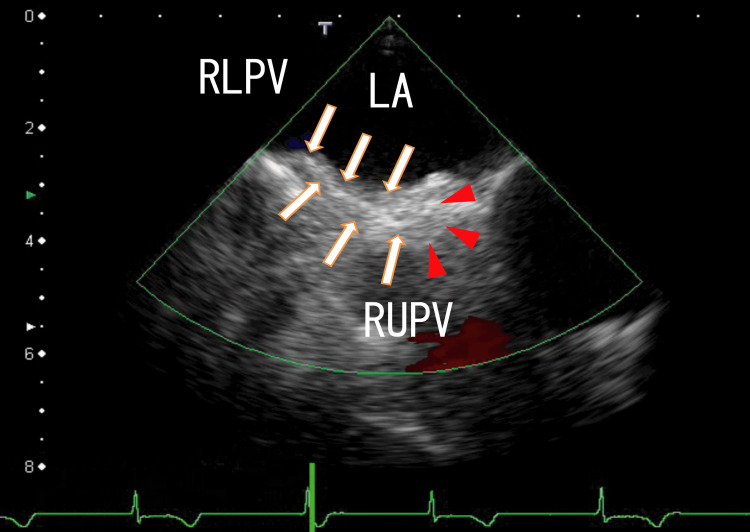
TEE images demonstrating that extended LA thrombi attached to the LA TEE images demonstrate thrombi in the LA as a line-like broad white mass. The inner side of the mass had extended and was attached to the right side of the LA anterosuperior wall near the entrance of the RUPV (arrows). The attached area is dim (arrowheads). The thrombi included a white area and were linearly shaped. The border between the right side of the thrombi and the LA wall was also vague. TEE, transesophageal echocardiography; LA, left atrium; RLPV, right lower pulmonary vein; RUPV, right upper pulmonary vein.
